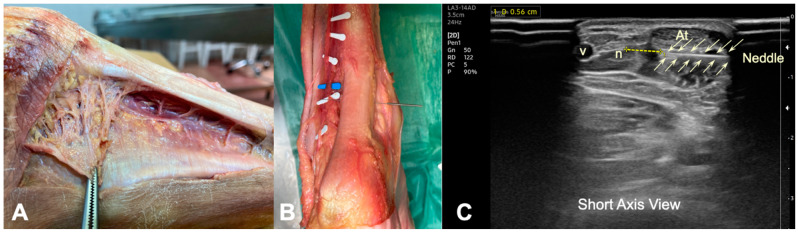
(A) Cadaveric view of the relationship between Kager’s fat and the deep face of the Achilles tendon. (B) Cadaveric detailed view of the needling insertion at the interphase between the Achilles tendon and Kager’s fat at 5 cm from the insertion in the calcaneus. (C) Ultrasound image (short axis view) of the measurement from the tip of the needle to the sural nerve inserted at the Kager’s fat–Achilles tendon interphase (from medial border) measured 5 cm from the insertion in the calcaneus. (At)—Achilles Tendon, (n)—Sural nerve, (v)—lesser saphenous vein.
